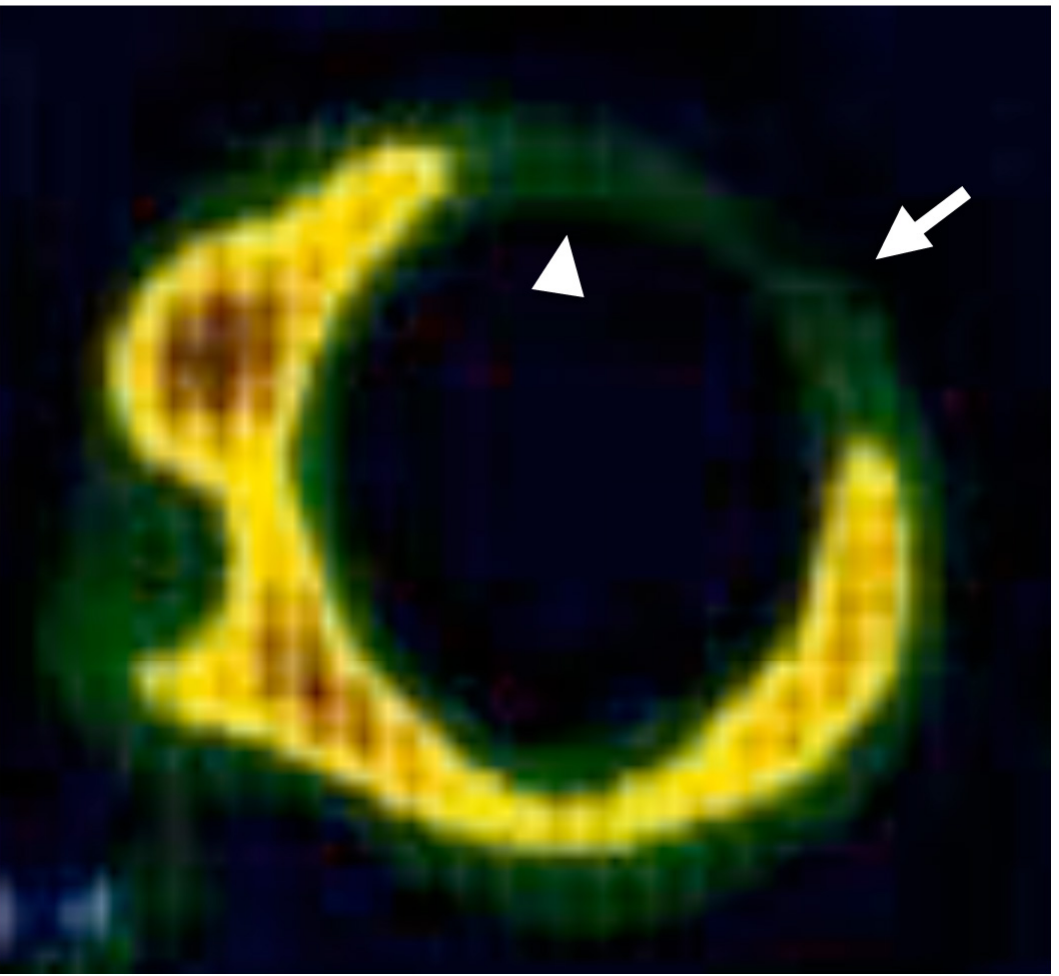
^201^Tl uptake was decreased from the anterior part of the IVS and anterior wall of the LV on cardiac scintigraphy. (Arrow: Anterior wall of LV, Arrowhead: Anterior part of IVS).
